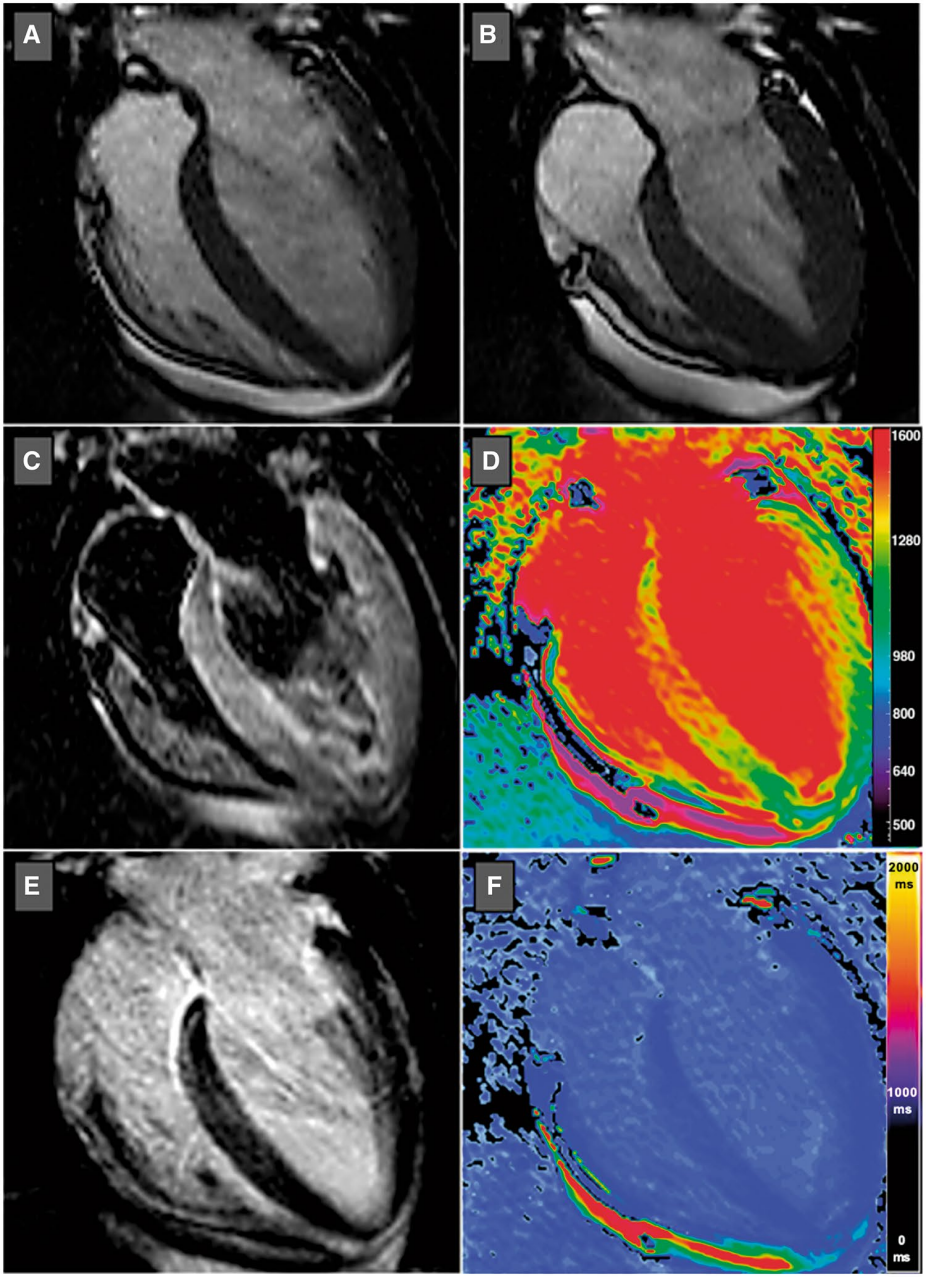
T1 mapping in takotsubo syndrome. End-diastolic (a) and end-systolic (b) balanced steady-state free precession cardiac magnetic resonance images in horizontal long-axis view showing basal and mid-ventricular hypokinesis and apical hyperkinesis compatible with reversed/basal takotsubo syndrome. Horizontal long-axis views of T2-weighted short tau inversion recovery (c) and late gadolinium enhancement (e) images revealed subtle hyperintensity and patchy contrast enhancement in the basal and mid-ventricular segments of the left ventricle. Colour-coded native (d) and post-contrast (f) T1 maps using shortened modified Look-Locker inversion recovery (ShMOLLI) confirmed regional myocardial involvement
